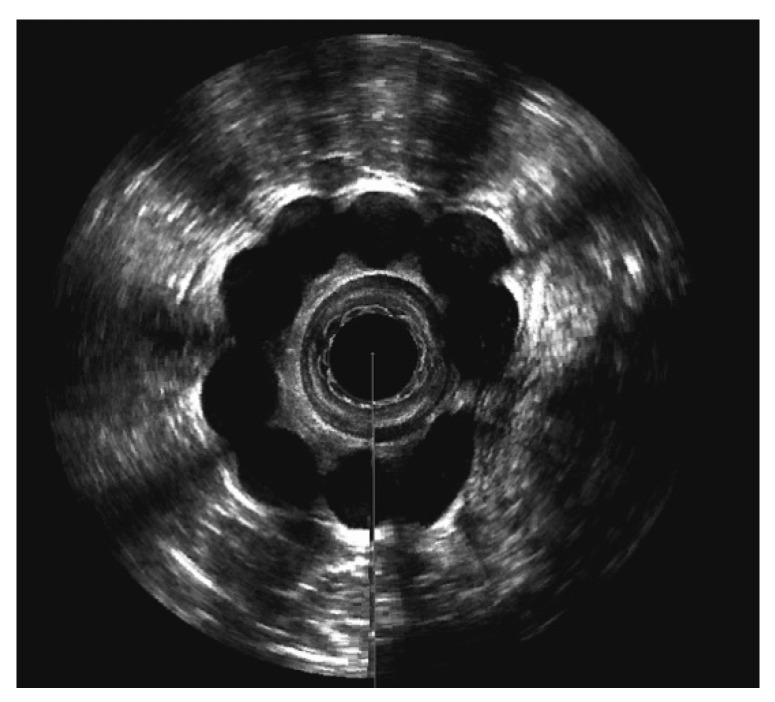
3D-endoanal ultrasound image of a patient at follow-up with nine Sphinkeeper prostheses.
